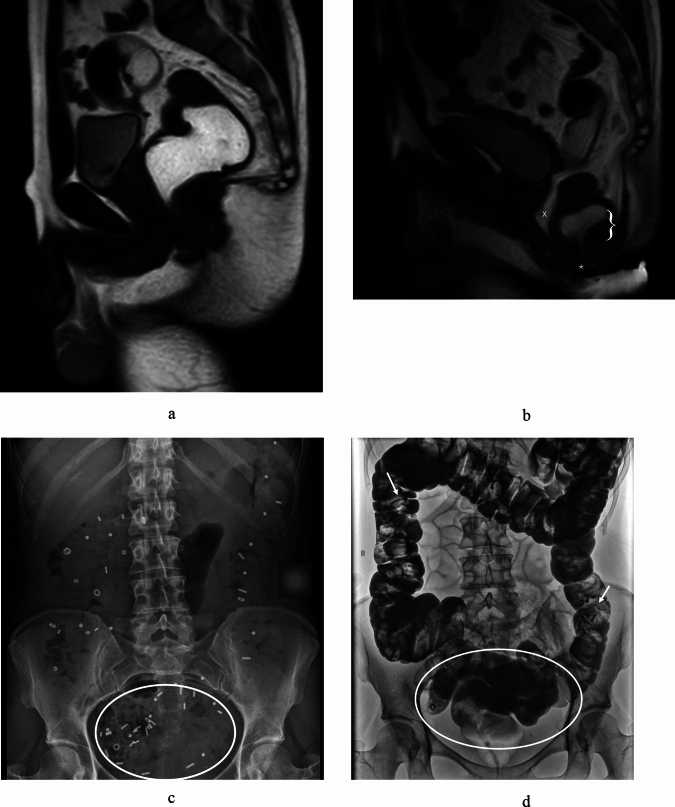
Representative radiologic images of the diagnostic work up for ODS. Magnetic resonance image defecography (MRI-D) in sagittal view of a male pelvis showing (A) the situation at rest and (B) at the end of defecation with a rectal prolapse (marked with *), the signs of the descending peritoneum as in a pelvic organ prolapse (marked with X), and intussusception of the distal rectum (marked with parenthesis “}”) .The X-ray of a colonic transit time (C) with distribution of the opaque markers in the ascending colon and the rectosigmoid (circled with a white ellipse). Image of the corresponding colon enema (D) showing an elongated rectosigmoid (circled white ellipse) and some residual markers from the colonic transit time examination 5 days and a bowel preparation later (white arrows).
